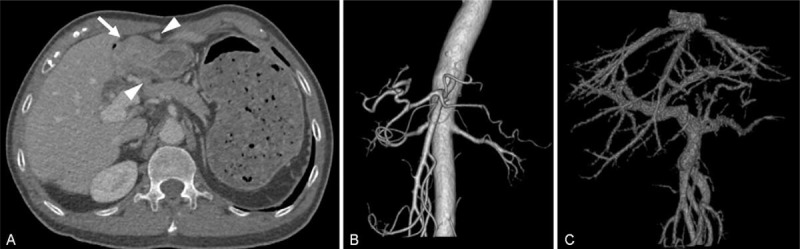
Images of 3-dimensional computed tomography (A, thickened antral wall (*arrow*) with multiple enlarged perigastric lymph nodes (*arrow head*) and no recurrence of hepatocellular carcinoma and distal metastasis were identified. B, There was no gross arterial anomaly around the stomach and hepatoduodenal ligament. C, The portal vein and its right branch were slightly dilated).
